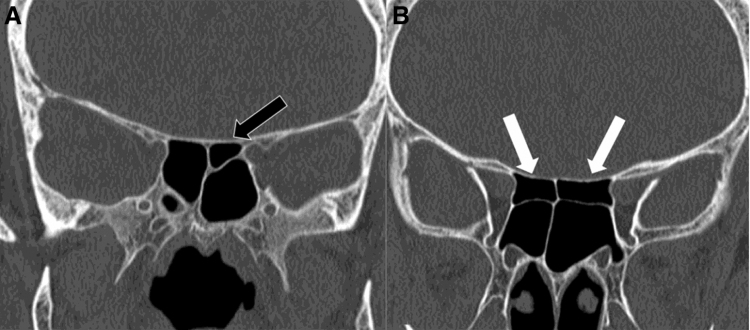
Coronal CT scan showing an Onodi cell (sphenoethmoidal cell, indicated by the arrow) superior and lateral to the sphenoid sinus. The presence of such a cell can alter the surrounding anatomy and is associated with a higher sphenoid ostium position.
